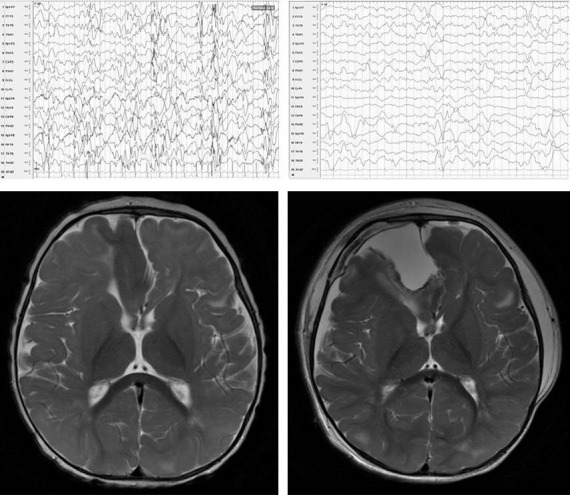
Upper left: hypsarrhythmia with predominant frontal spikes, lateralized to the right. Upper right: Absence of hypsarrhythmia with right frontal slowing and right frontal sharp waves. Lower left: MRI brain showing presence of large right frontal cortical tuber. Lower right: MRI brain following tuber removal.
